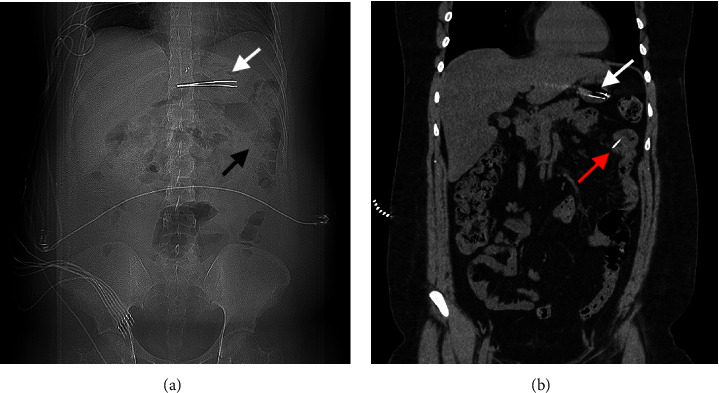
(a, b) Scout CT image showing both FB in the stomach (white arrow) and the small bowel (black arrow), respectively. Coronal CT scan showing both objects in the stomach (white arrow) and the small bowel (red arrow).
